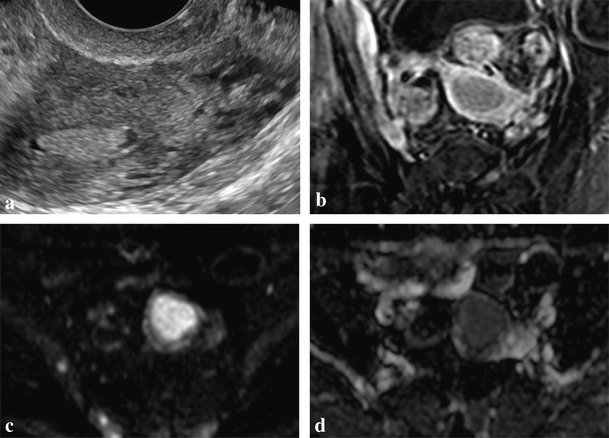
a–d. Mixed serous-endometrioid carcinoma of the uterus in a 66-year-old woman with Lynch syndrome. Ultrasound (a) shows a solid polypoid mass inside the uterine cavity and a small hydrometra. MRI scan demonstrates a solid well-circumscribed endometrial tumour, hypoenhancing compared with myometrium on post-gadolinium FS T1-weighted image (b). Diffusion restriction on the b1000 image (c) with a low apparent diffusion coefficient (ADC) value (d) is also evident, consistent with its malignant nature. The tumour was confined to the corpus uteri with no myometrial invasion
